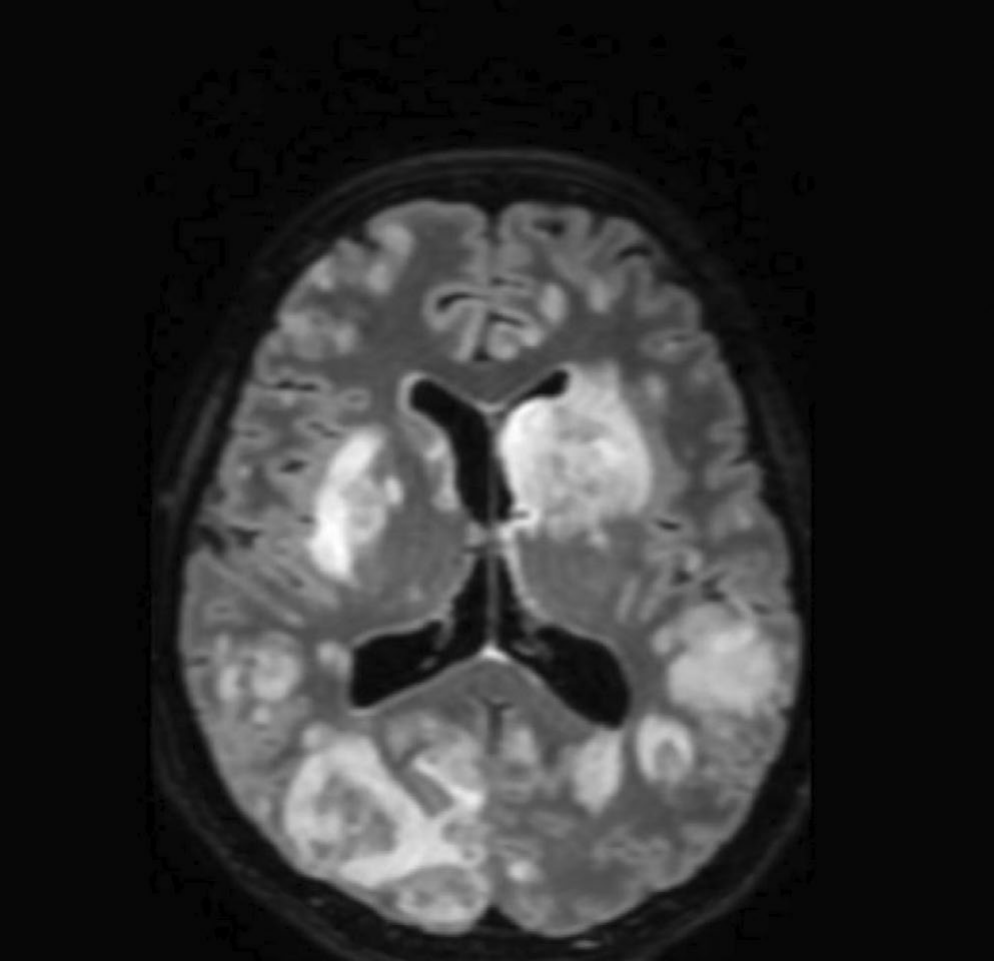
FIGURE 2 CASE 1 Multiple FLAIR‐hyperintense lesions, with the concentric target sign seen in the deep parenchymal lesions.
